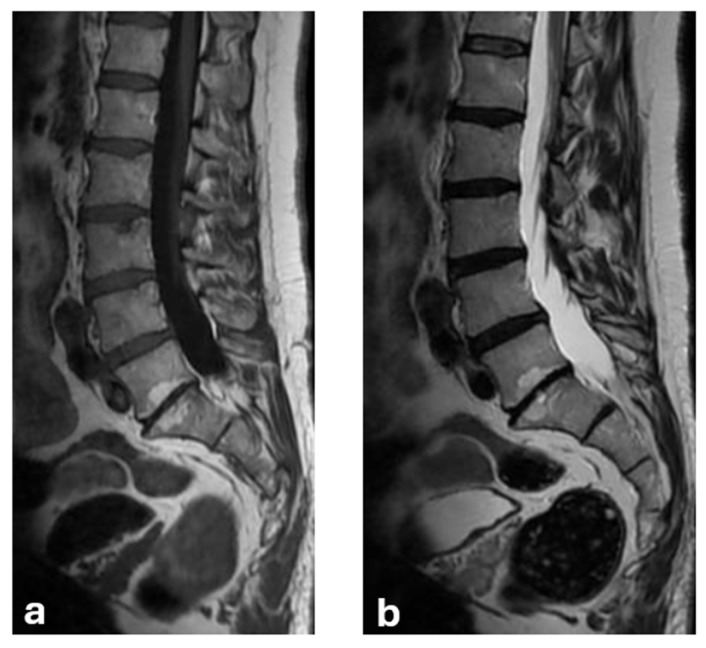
Type 2 Modic change. (a) T1-weighted. (b) T2-weighted.
